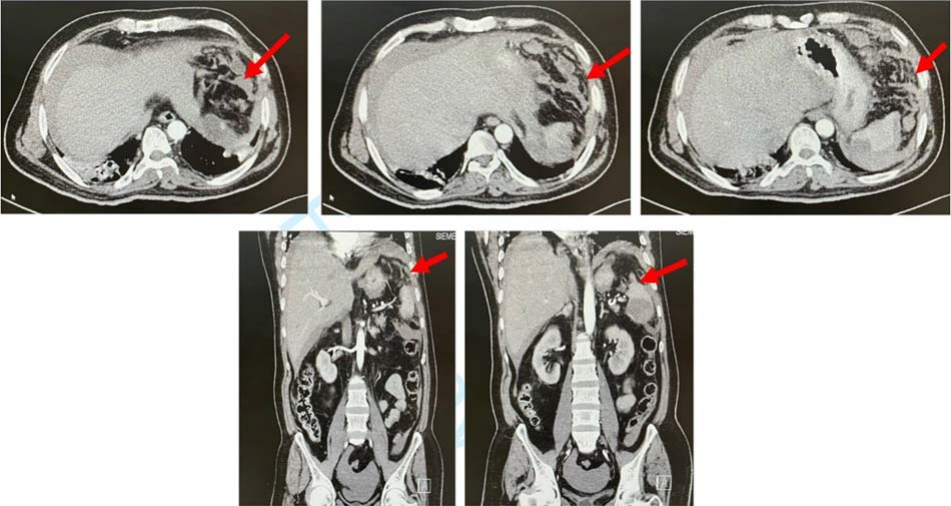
CT abdomen. Axial and coronal views showing splenic rupture and hemoperitoneum (red arrow).
